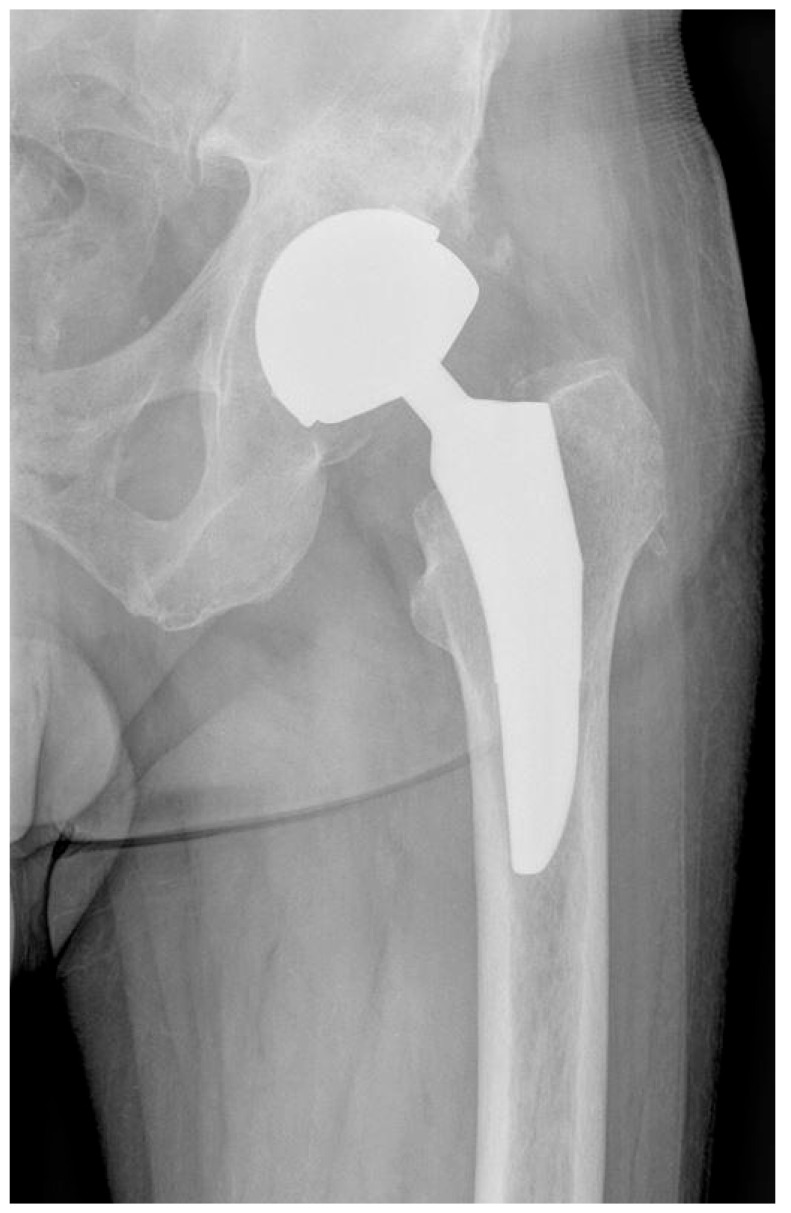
X-ray scan showing the hip operated with use of ReCap-Magnum with a Microplasty metaphyseal stem (Biomet, Warsaw, IN, USA).
